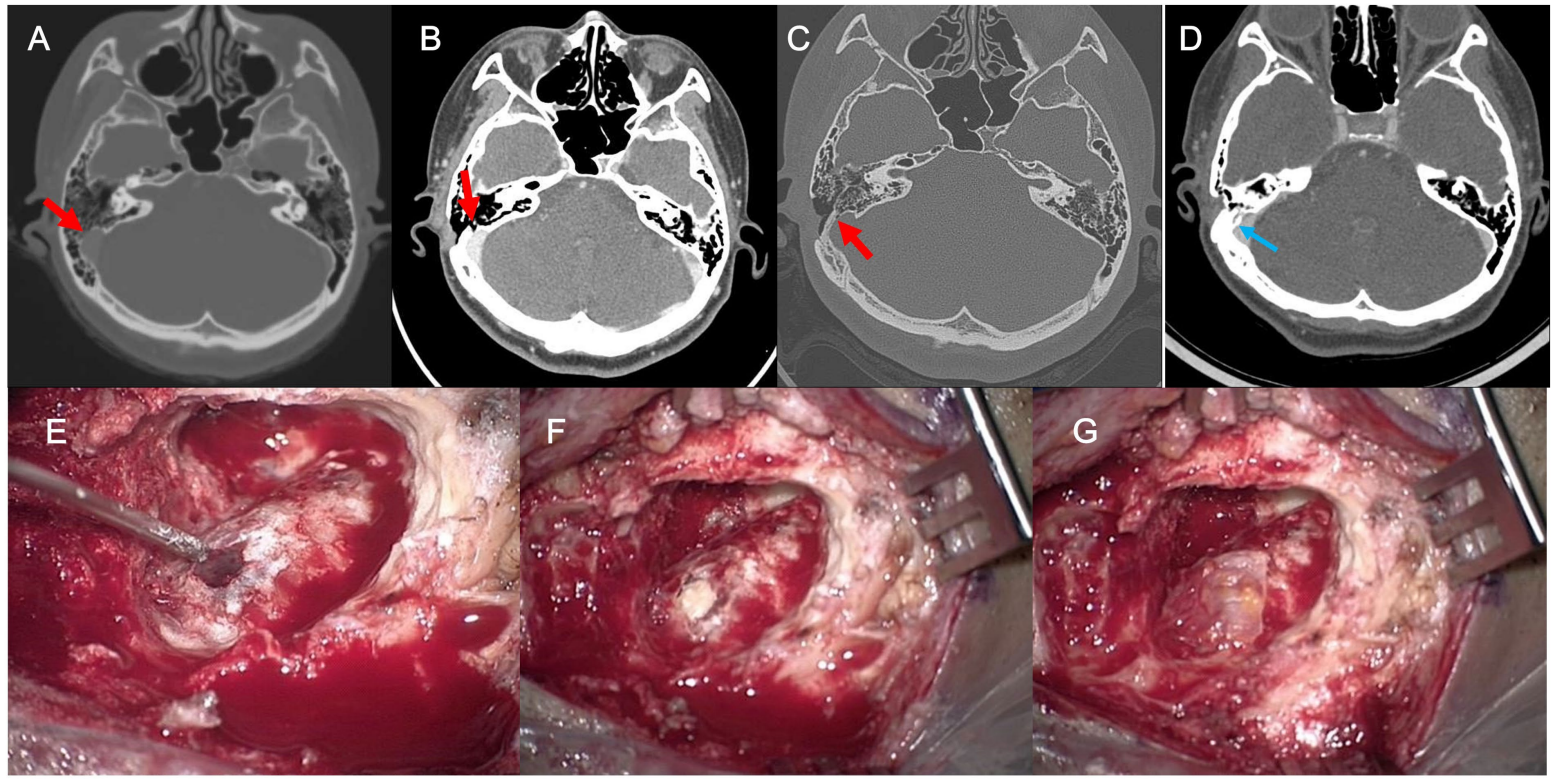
(A) Preoperative axial temporal bone computed tomography (TBCT) image of Subject 3 shows a diverticulum protruding through the mastoid air cells with bony dehiscence (arrow). (B,C) Follow-up postoperative TBCT axial image with and without contrast enhancement shows a residual bony dehiscence (arrow). (D) The dehiscence is successfully managed by transmastoid reshaping with a cortical bone chip, a piece of temporalis fascia, and bone cement (arrow). (E) The residual bony dehiscence was exposed. (F,G) Revision reshaping of the sigmoid sinus diverticulum with dehiscence was performed with a cortical bone chip, a piece of temporalis fascia, and bone cement to reconstruct a secure sinus wall.
